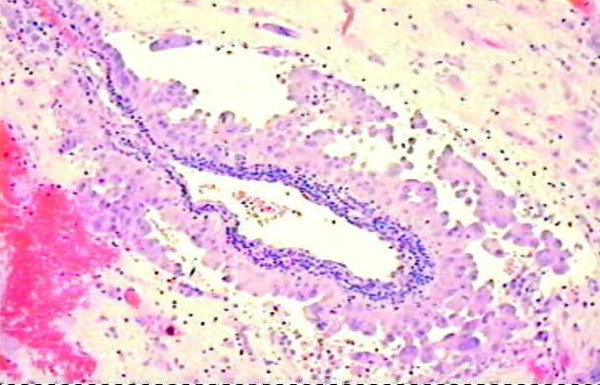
CT guided biopsy of adrenal lesions, showing malignant adenocarcinoma cells.
